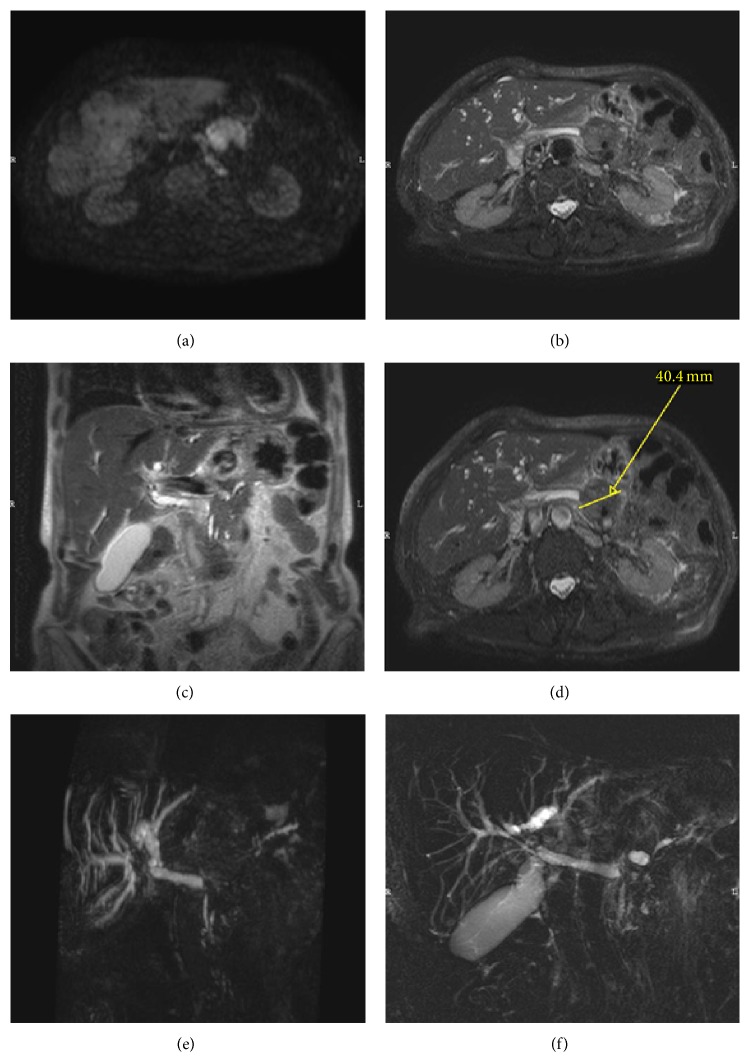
NMR evaluation of pancreatic lesion. (a) Diffusion weighted imaging (DWI) axial sequence shows a hyperintense pancreatic body mass with restricted apparent diffusion coefficient (ADC) value, confirmed in ADC map; (b) single shot axial T2-weighted fat sat image shows pancreatic body mass isointense to liver parenchyma; (c) single shot coronal T2-weighted image shows dilated extrahepatic biliary duct; (d) as shown in (b), with the indication of diameter of the lesion (4 centimeters); (e) 3D maximum intensity projection (MIP) coronal image showing dilated intra- and extrahepatic biliary ducts without evidences of middle segment of Wirsung duct; (f) T2-weighted radial big slab image of the same condition presented in (e).
